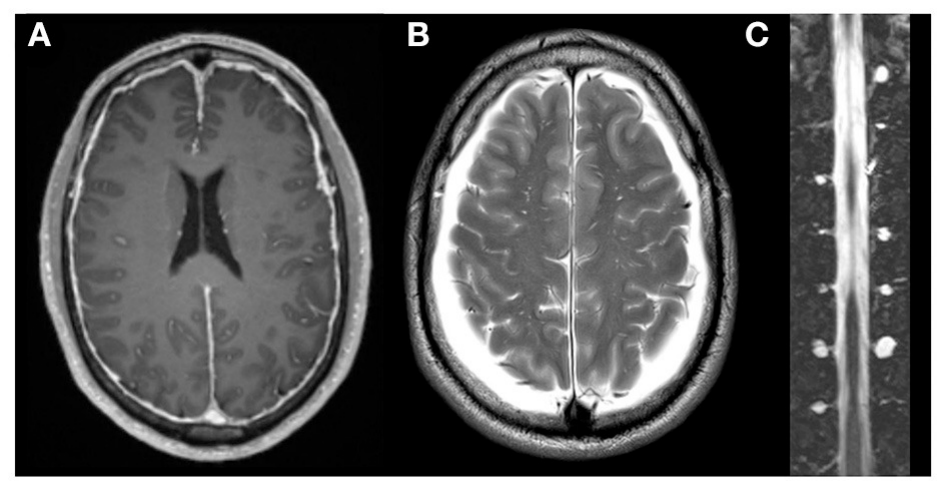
Imaging of a patient with SIH symptoms without evidence of CSF leakage or CSF venous fistula. A 68-year-old male patient exhibiting classic SIH symptoms with typical cranial imaging findings, such as dural enhancement (A) and bilateral subdural hygroma (B) and no evidence of CSF leakage or CSF venous fistula despite repeated diagnostic work-up. Spinal MRI revealed a large number of prominent meningeal diverticula (C).
